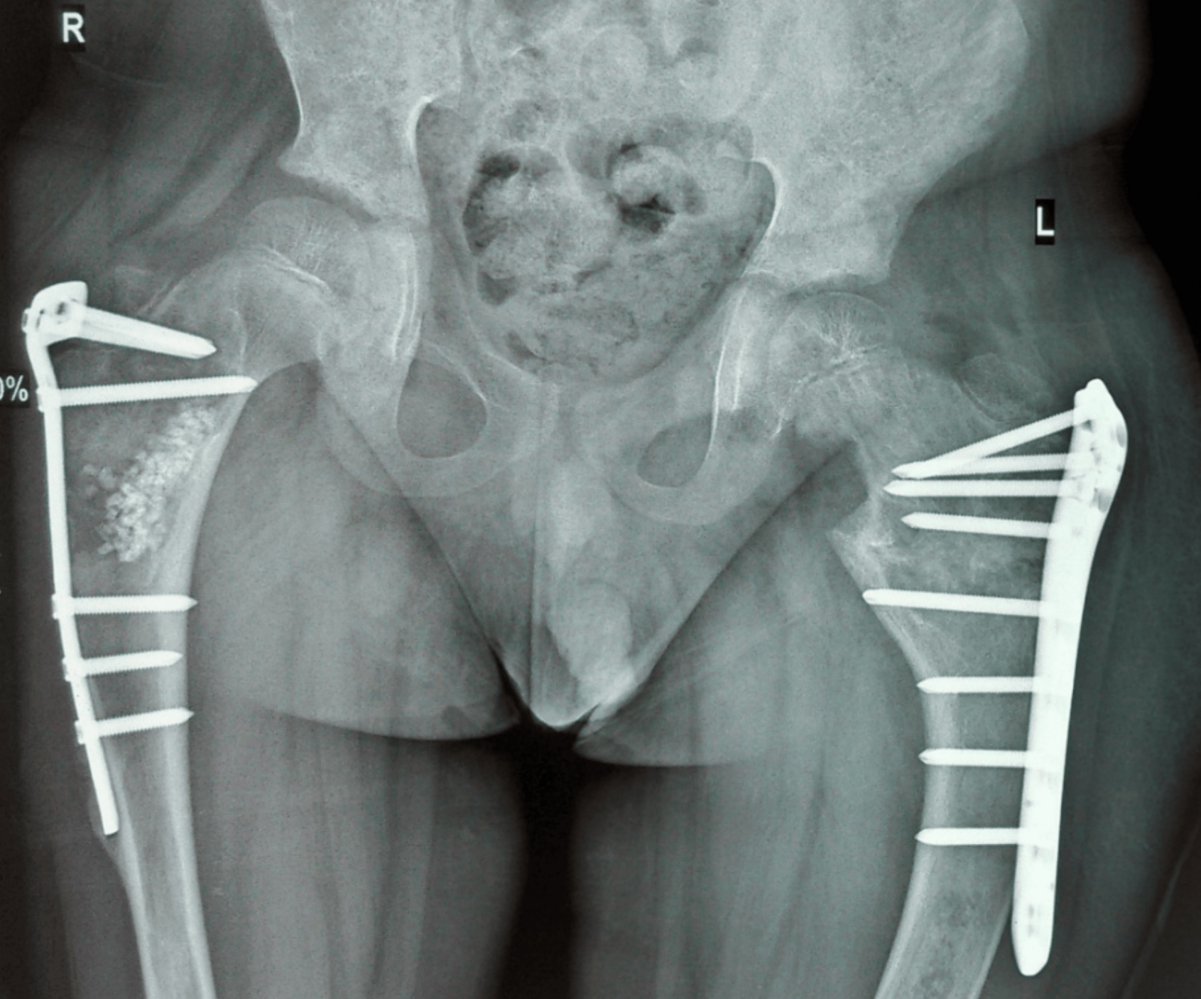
X-ray showing valgus osteotomy with open reduction and internal fixation and bone grafting
